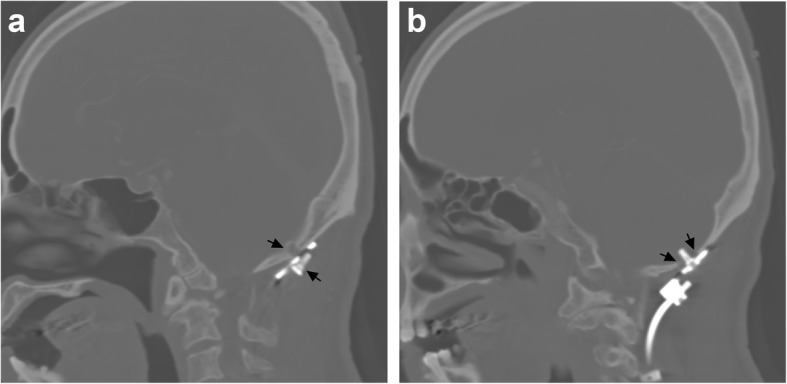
CT sagittal images of the failure of the OC fusion instruments. (a) The arrow showed the dislodgement of the occipital screw and the screw hole. The screw was loose and not in their original position. (b) The arrow indicated the oversized diameter of the screw hole. The diameter of the screw hole is much larger than the size of the screw, indicating that the screw has no fixing effect
